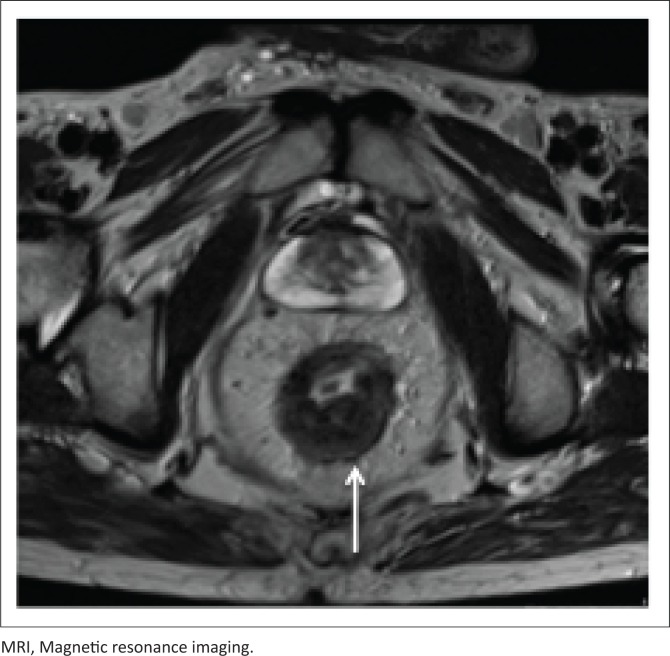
Axial T2-weighted MRI imaging. The patient is a 50-year-old male with thickening of the posterior wall of the rectum. MRI was performed to aid surgical management.
